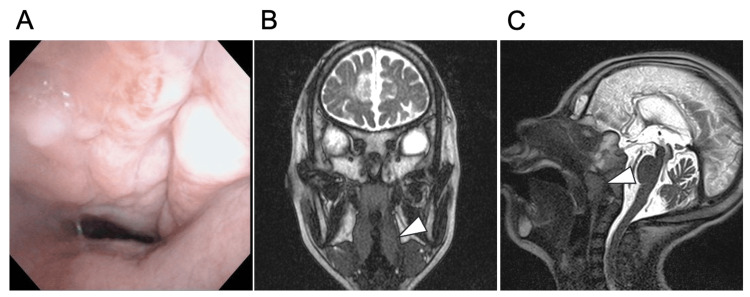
Physical examination and findings of patient 2 A: Nasopharyngeal fiber scopy showed pharyngeal stenosis, particularly at the level of the oropharynx. B and C: MRI (B: coronal section, C: sagittal section) shows tonsil and adenoid hypertrophy (white triangle).
